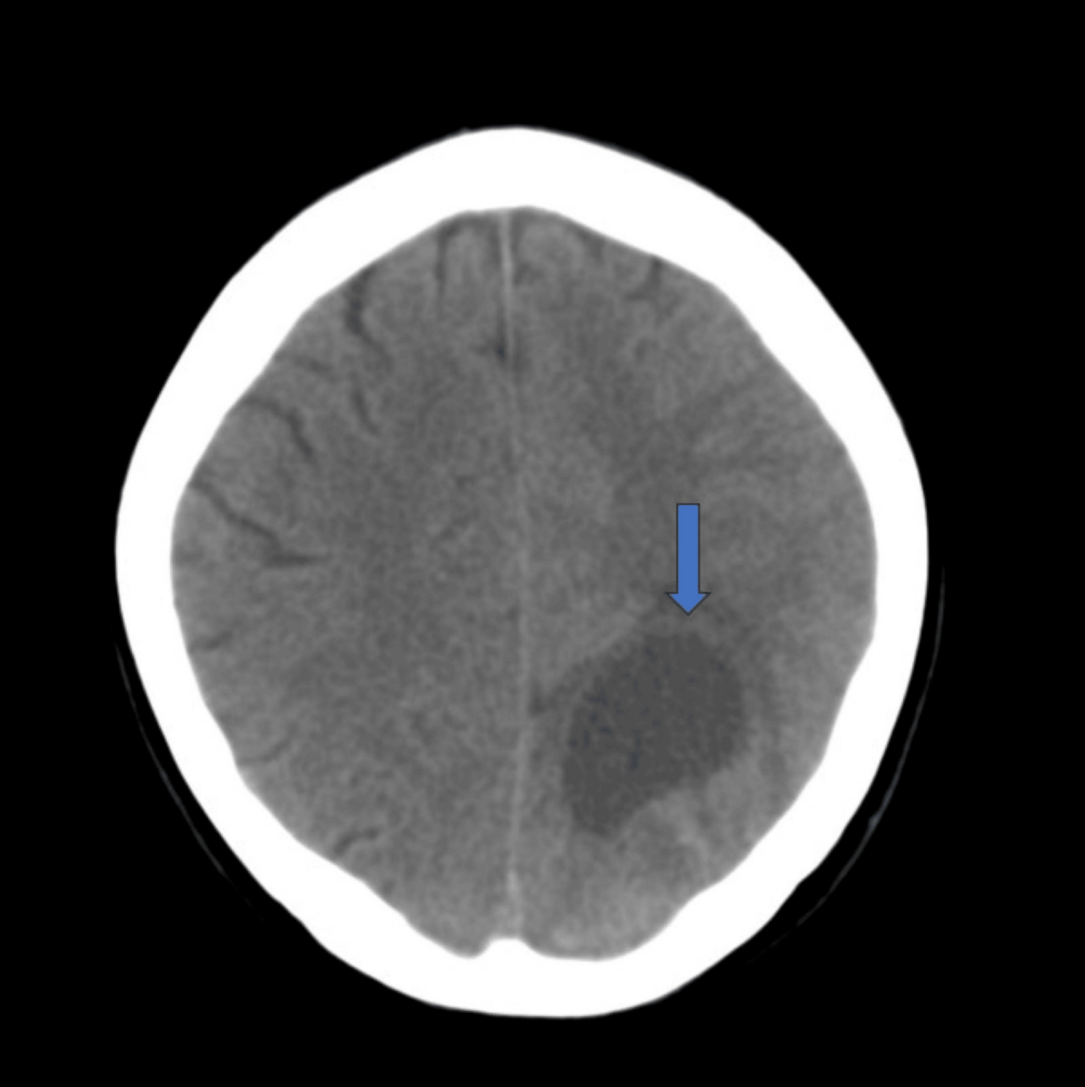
CT of the head showing a 4.5 cm rim-enhancing cystic lesion in the left occipital lobe and extensive surrounding edema and mass effect along with a left middle cerebral artery (MCA) bifurcation aneurysm
